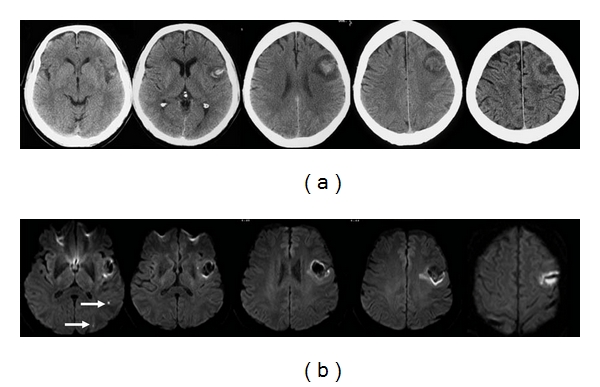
60-year-old man presented with dysphasia and confusion for 2 days. (a) Axial unenhanced CT images show a left frontal hematoma with surrounding hypodensity spreading from the centre, reaching superiorly and out to the cortical surface. The shape and topography of the lesion suggest that the primary event is an infarct, with secondary hemorrhagic transformation. Stage HI c2 [1]. (b) Axial diffusion weight MR images show 2 small discrete lesions within the left parietal and temporal lobes, suggesting concurrent infarcts in the same arterial territory. This further supports that the initial lesion is a hemorrhagic infarct, probably embolic in nature.
